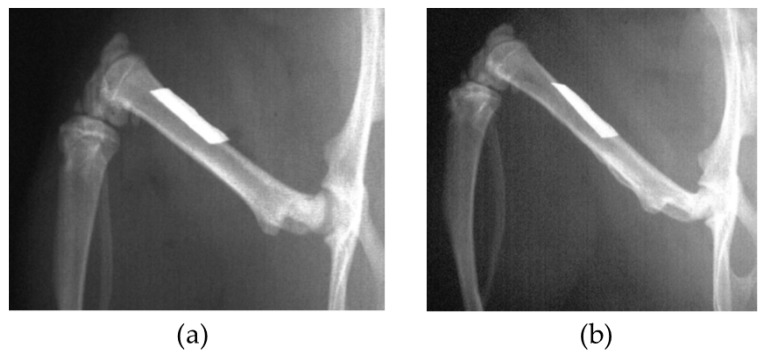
(a) Radiograph of left femoral bone just after BMG ribbon implantation; (b) Radiograph of femoral bone and BMG ribbon at 6 weeks after implantation. The size and shape of the ribbon have not changed from the original ones.
